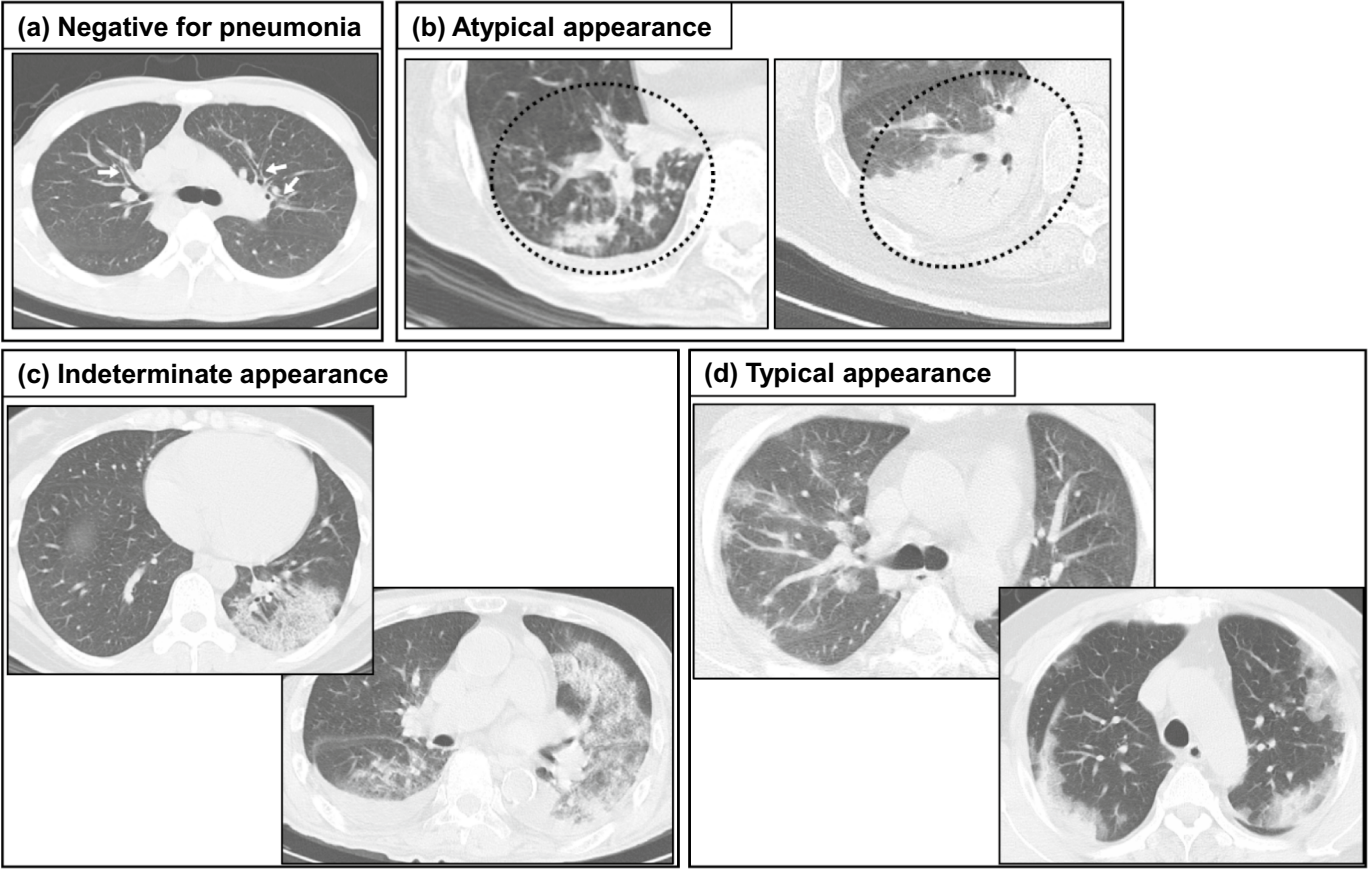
Example of chest CT patterns of RSNA classification. Axial CT images are categorized as (a) “Negative for pneumonia” meaning no features of pneumonia, (b) “Atypical appearance”, meaning typical for other infection but not COVID-19, e.g., bronchial pneumonia, lobar pneumonia, tuberculosis, or fungal infection, (c) “Indeterminate appearance”, meaning the presence of feature suspicious for COVID-19 but with overlaps with other diseases, drug-induced pneumonia, collagen disease-related lung diseases, or alveolar pulmonary edema, and (d) “Typical appearance”, meaning the typical pattern of COVID-19 pneumonia [49]
